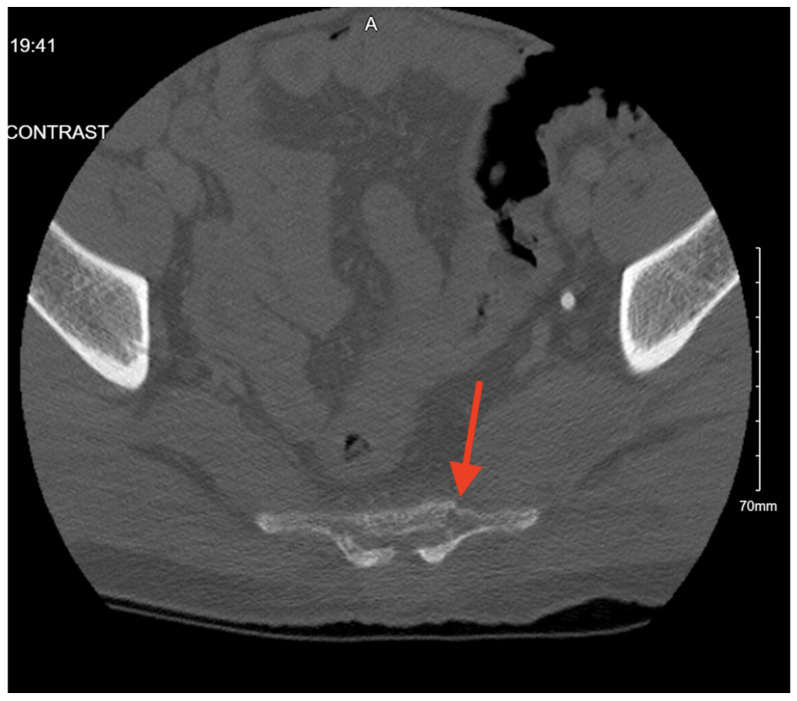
CT abdomen/pelvis images of the described fracture in case 4. Red arrows are used to indicate the location of pelvic fractures for easier identification.
